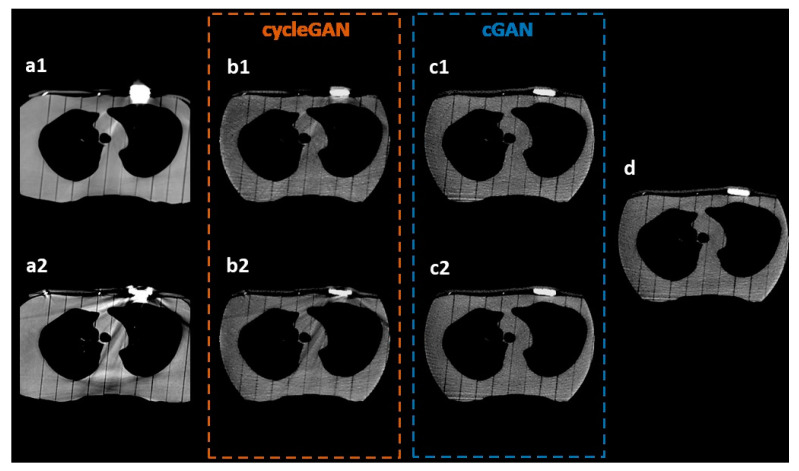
Visual inspection of the artifact correction in synthetic images. The synthetic images generated by different models were compared with the ground-truth real MV CBCT images. Axial views of (a1, a2) original CT or kV CBCT images, (b1, b2) synthetic images generated by cGAN models, (c1, c2) synthetic images generated by cycleGAN models, and (d) ground-truth MV CBCT image.
